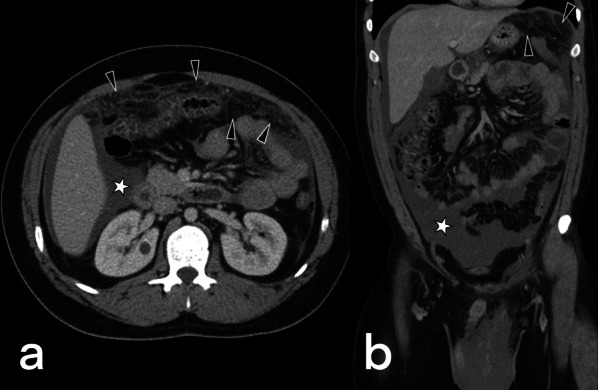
A 24-year-old male patient. Axial (a) and coronal postcontrast CT (b) images show mesenteric striation (arrowheads) and ascites (star)
